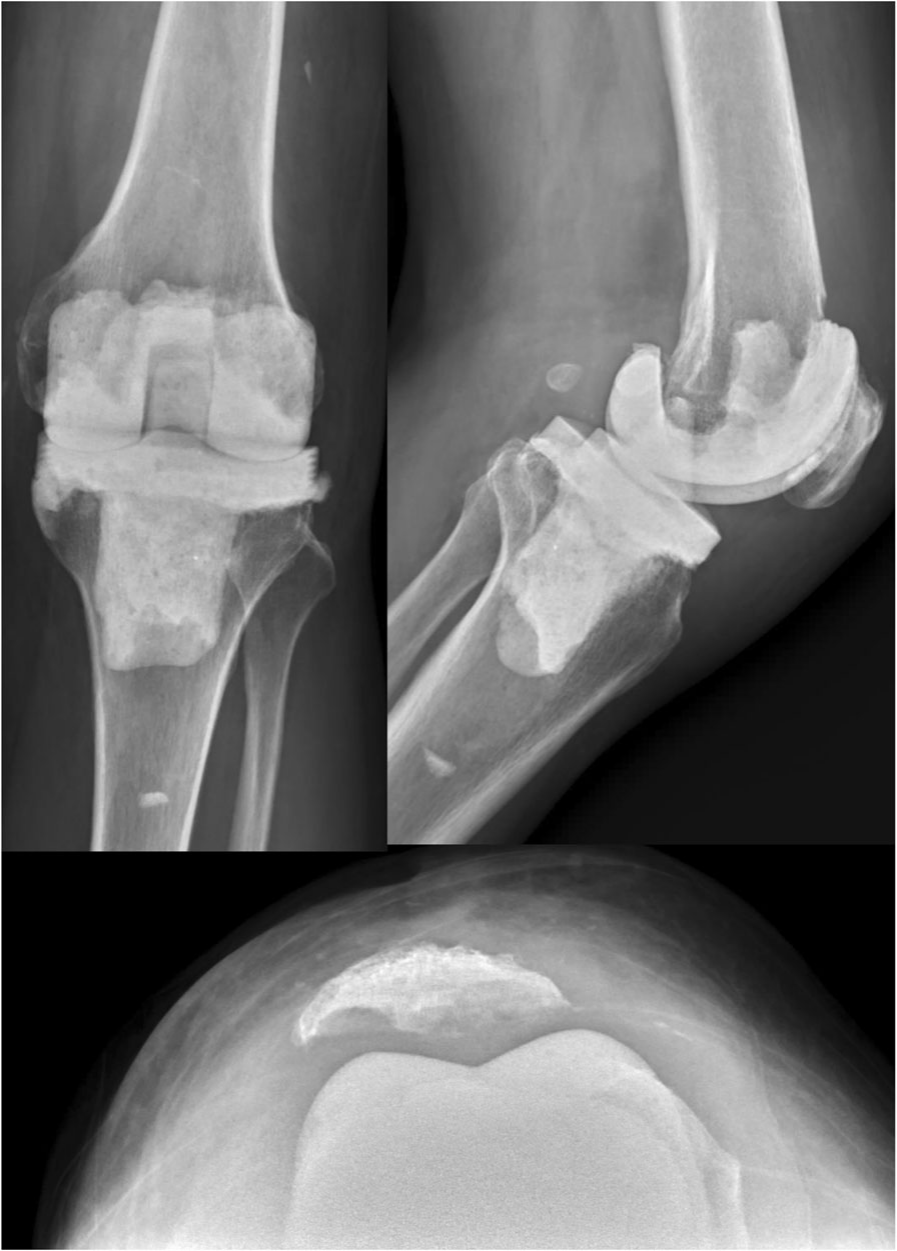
Knee anteroposterior, lateral, and skyline Merchant radiographs were taken with the CAD-articulating spacer in situ
